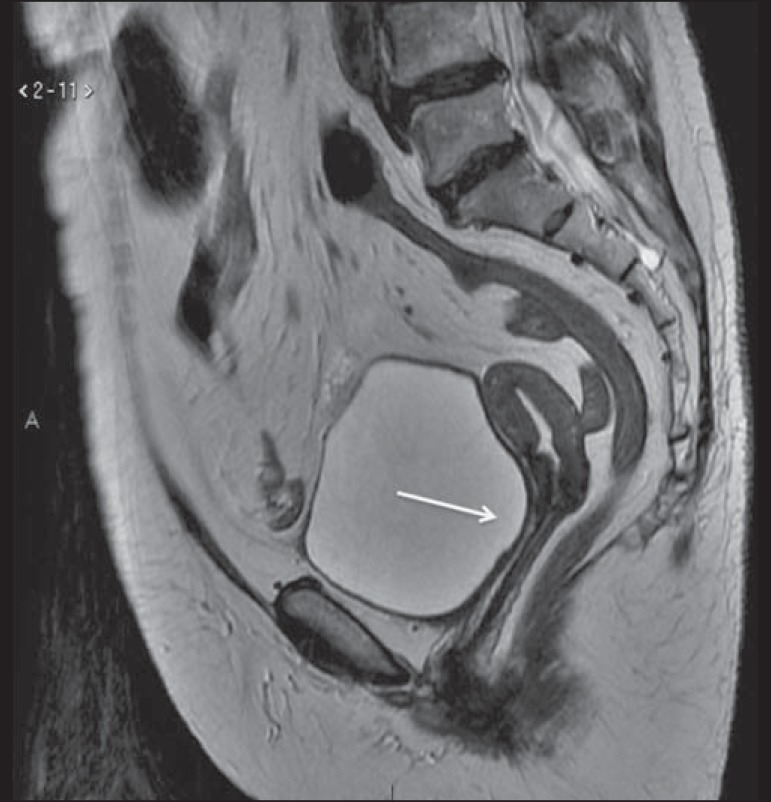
MRI scan requested for the assessment of pelvic tumor recurrence in a
